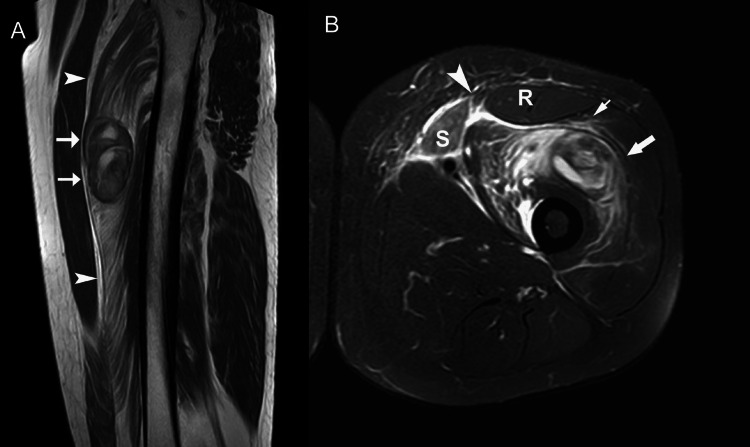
A 27-year-old male with a laceration anterior to the thigh. (A) Sagittal T1-weighted MR image through the thigh shows acute hematoma (arrows) with intermediate signal intensity in injured vastus intermedius muscle. Note the extensive hemorrhage infiltrating the muscle adjacent to hematoma (arrowheads). (B) Axial fat-suppressed fast spin-echo T2-weighted MR image reveals defect (arrowhead) in anterior musculature between sartorius (S) and rectus femoris (R) overlying hematoma (arrow) within enlarged vastus. Findings are consistent with muscle laceration with the formation of hematoma from a stab wound. Note perifascial edema (thin arrow).
